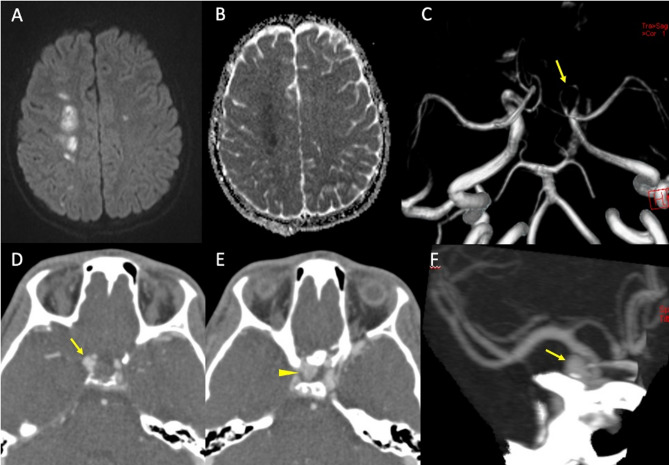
Blunt trauma in a patient at the beginning of the third decade of life involved in a motor vehicle accident. MRI (top row) shows areas of restricted diffusion (A: diffusion weighted imaging sequence and B: apparent diffusion coefficient map) in the centrum semiovale of the right cerebral hemisphere, consistent with acute ischemia. MRA (C) demonstrates distinct narrowing of the right intracranial carotid artery (arrow) involving the cavernous and supraclinoid segments, consistent with post-traumatic dissection. Follow-up CTA one month from presentation (D-F) shows the appearance of a bilobated post-traumatic aneurysm (Denver grade III) of the right internal carotid artery, with a component arising from the supraclinoid tract and directed supero-laterally (D, arrow) and a component arising from the cavernous portion and directed medially in the sellar region (E, arrowhead). Maximum intensity projection reconstruction (F) more clearly depicts the supraclinoid portion of the aneurysm (arrow)
